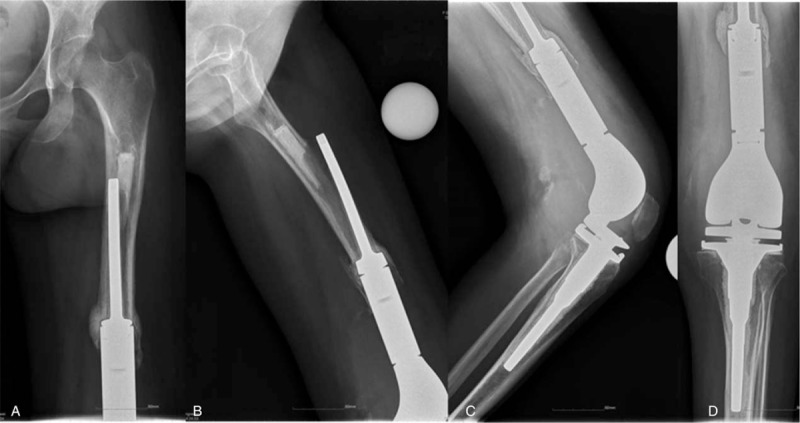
Patient's anteroposterior and lateral x-ray image. A, Anterior-posterior x-ray of femur. B, Medial-lateral x-ray of femur. C, Anterior-posterior x-ray of knee. D, Medial-lateral x-ray of knee.
